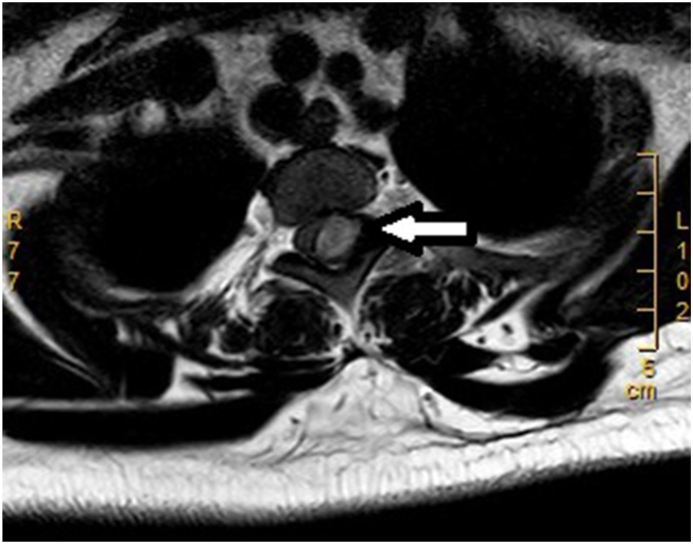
Thoracic MRI of the patient. Axial T3 sequence shows abnormally enhanced epidural signals (arrow), which pushed the spine to the right.
